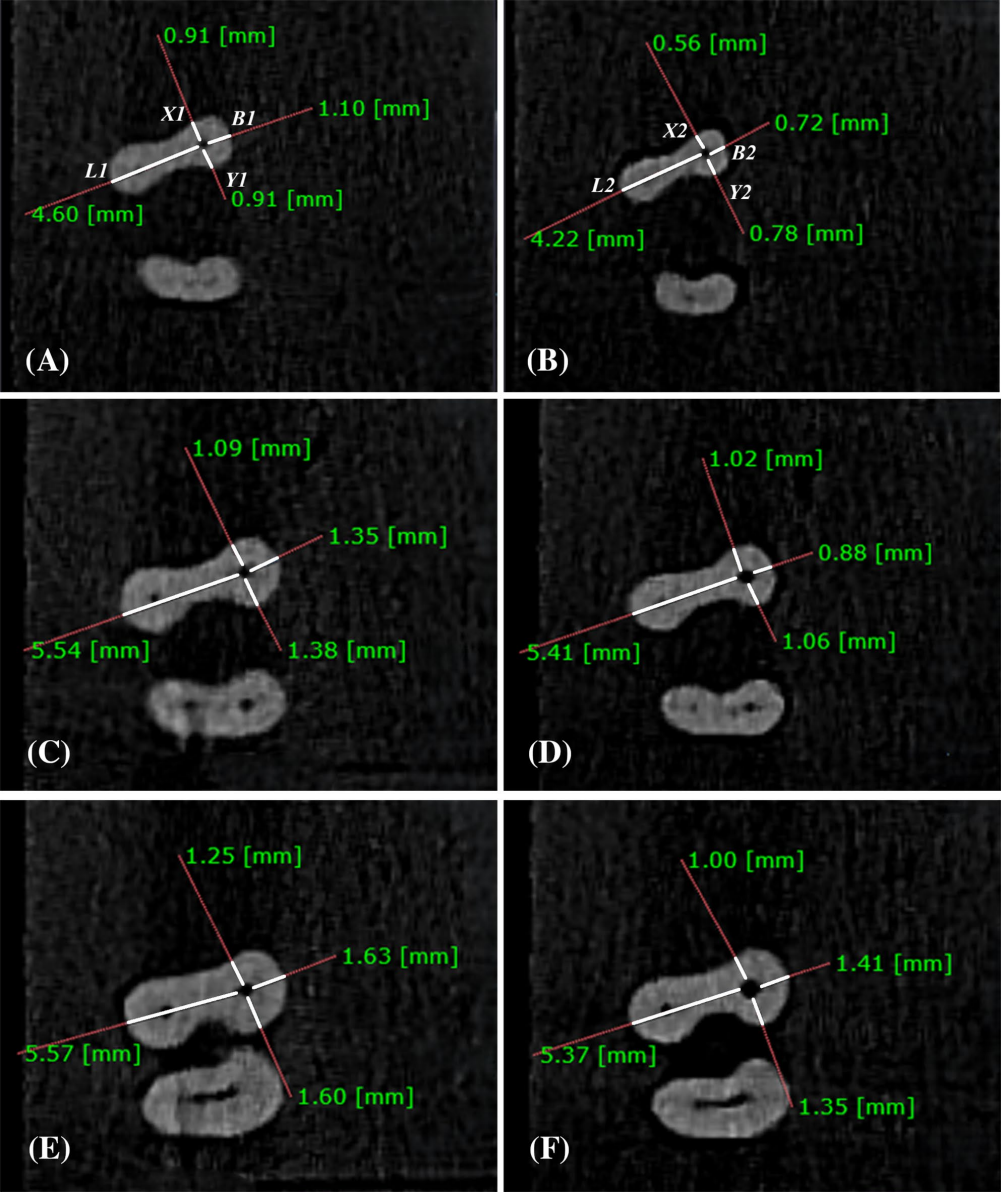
Showing CBCT images analysis of one of the samples at three, six- and nine-mm levels. A & B Axial sections of pre and post instrumentation measurement at three mm level, respectively. C & D Axial sections of pre and post instrumentation measurement at six mm, respectively. E & F Axial sections of pre and post instrumentation measurement at nine mm, respectively
